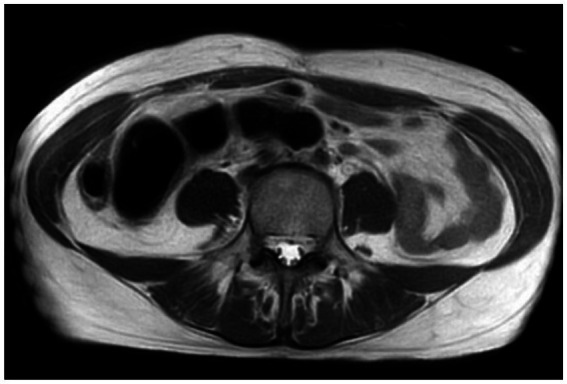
Pelvic MRI: No signs of occlusion.
